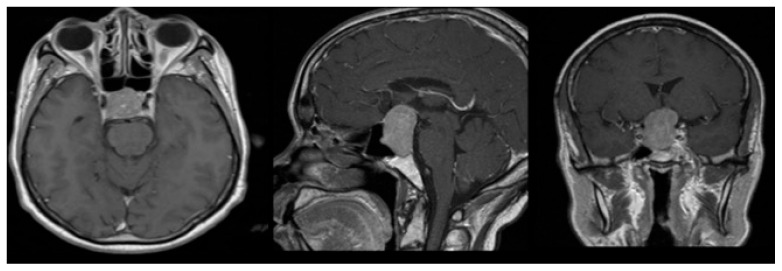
Axial, sagittal, and coronal MRI performed before surgery.
